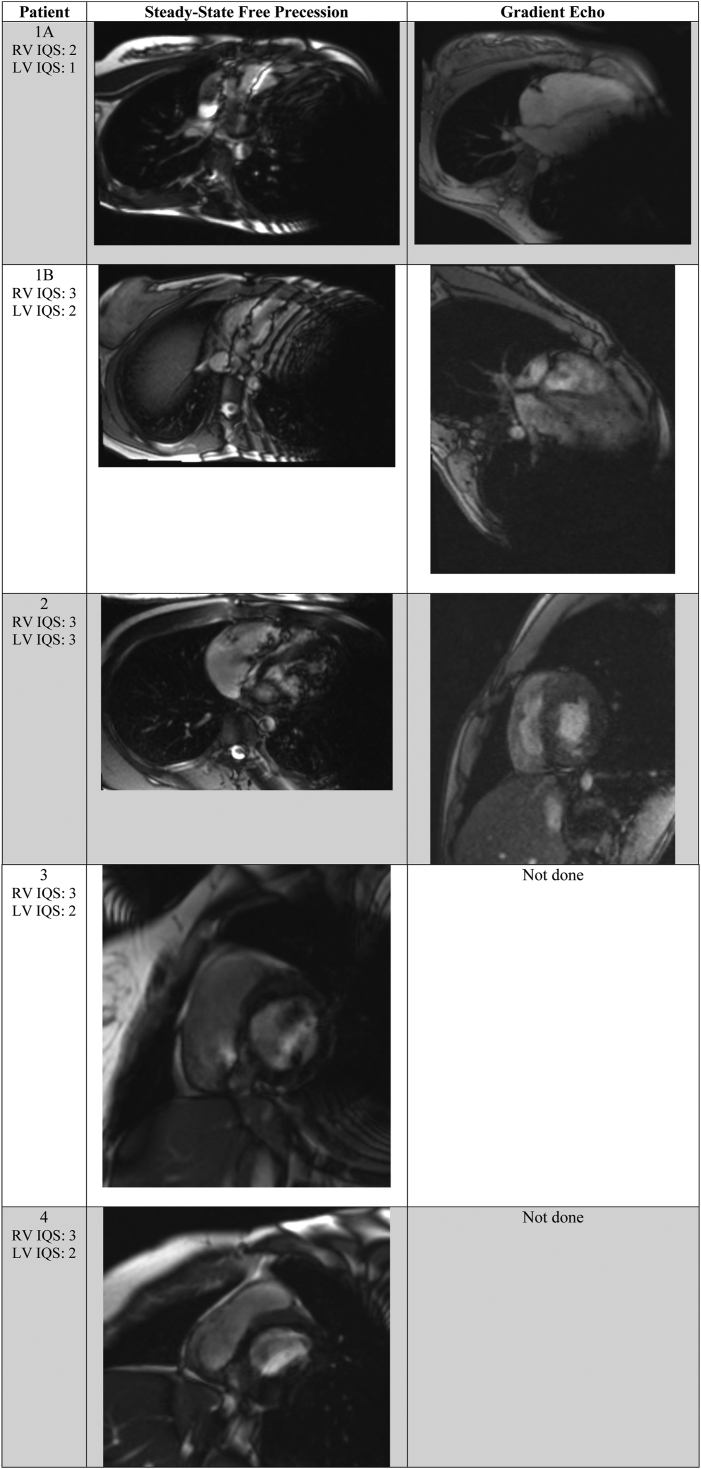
Image quality score (IQS) shown alongside corresponding cardiac magnetic resonance images, including steady-state free precession sequences and gradient echo sequences. LV = left ventricle; RV = right ventricle.
